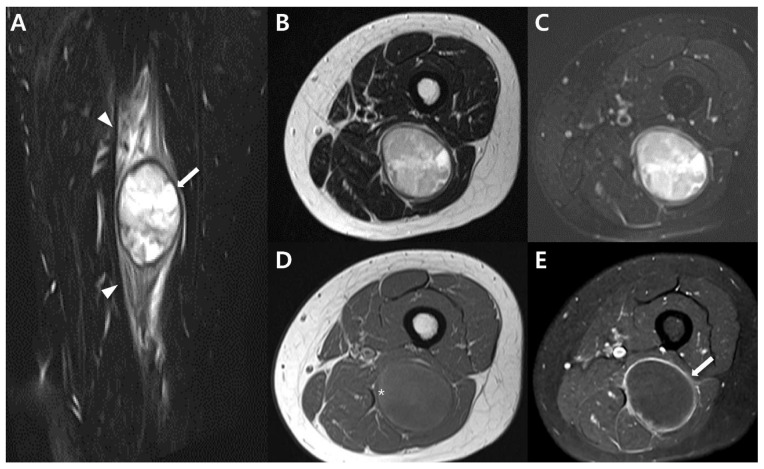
Intraneural synovial sarcoma of 37-year-old-women (monophasic type): (A) Coronal T2-weighted, fat-suppressed image shows about 4.3 × 4 cm sized oval circumscribed mass (arrow) along the sciatic nerve in posterior aspect of Lt thigh. Note extensive peritumoral edema (arrowheads). (B,C) Axial T2-weighted images without and with fat suppression show well-circumscribed mass between semimembranosus and semitendinosus muscle. The mass shows heterogenous signal intensity without typical target sign. (D) Axial T1-weighted image shows iso to high (*) signal intensity compared to adjacent muscle. High (*) signal intensity region suggests hemorrhage component. (E) Axial T1-weighted post-contrast image shows peripheral rim enhancement (arrow), suggesting totally cystic change.
